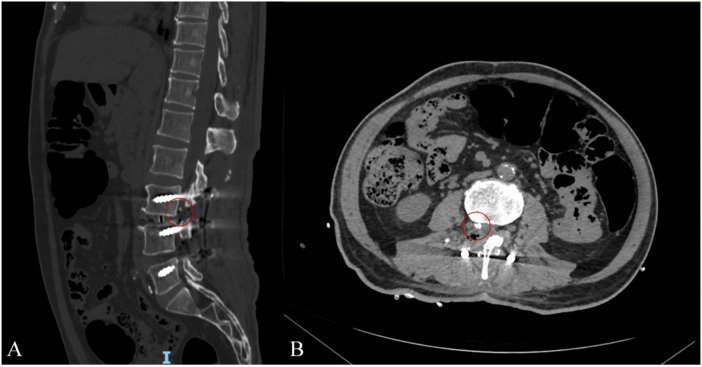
(A) Shows the lumbar CT on the sagittal view indicating residual bone mass continuously compressing the nerve roots, while (B) is its axial view.
